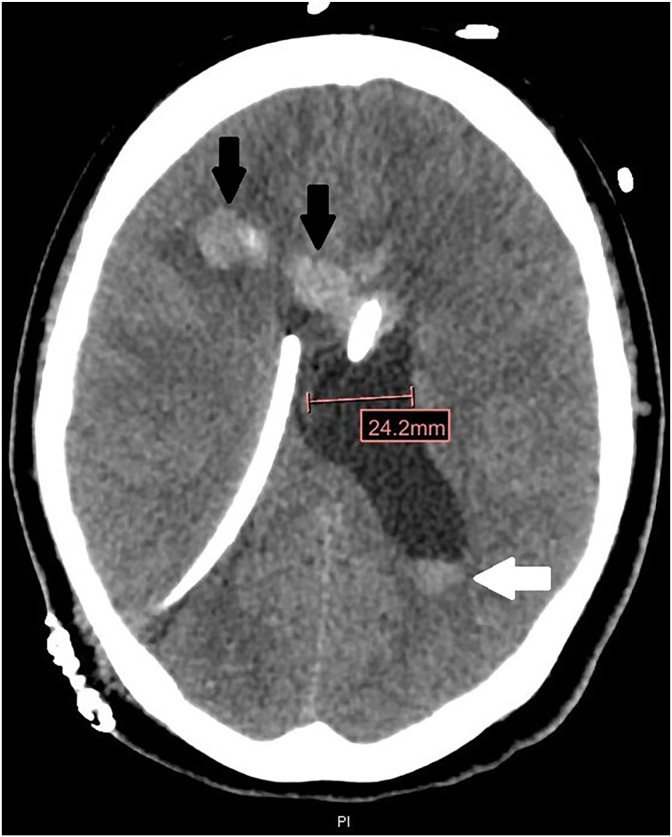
Computed tomography of the head on Day 22 shows dilated left lateral ventricle with parenchymal hemorrhage in the right frontal lobe (black arrows) and intraventricular hemorrhage (white arrow) despite ventriculostomy tubes.
